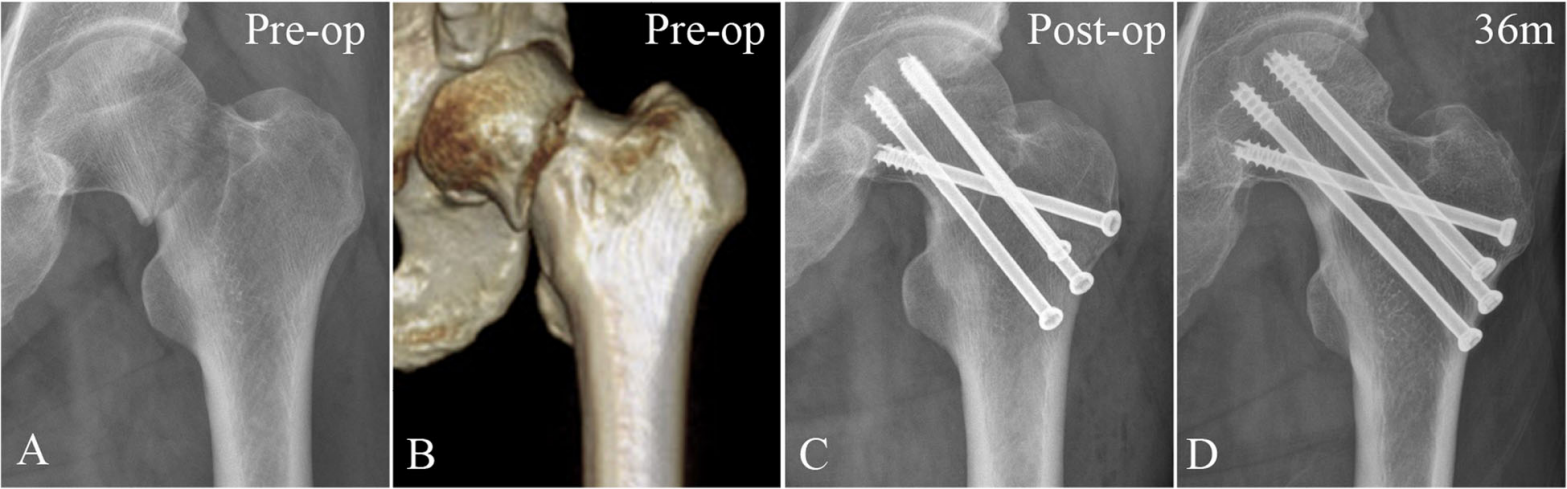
3-D reconstructed model of hip and AP radiographs of fracture and treatment fixation in a 49-year-old male with a vertical femoral neck fracture. a, b Preoperative radiograph and reconstructed model showing the vertical femoral neck fracture. c AP radiograph showing the initial treatment with three parallel screws augmented with an off-axis screw. d AP radiograph of the same patient taken 36 months postoperatively, revealing bone union without any complications
